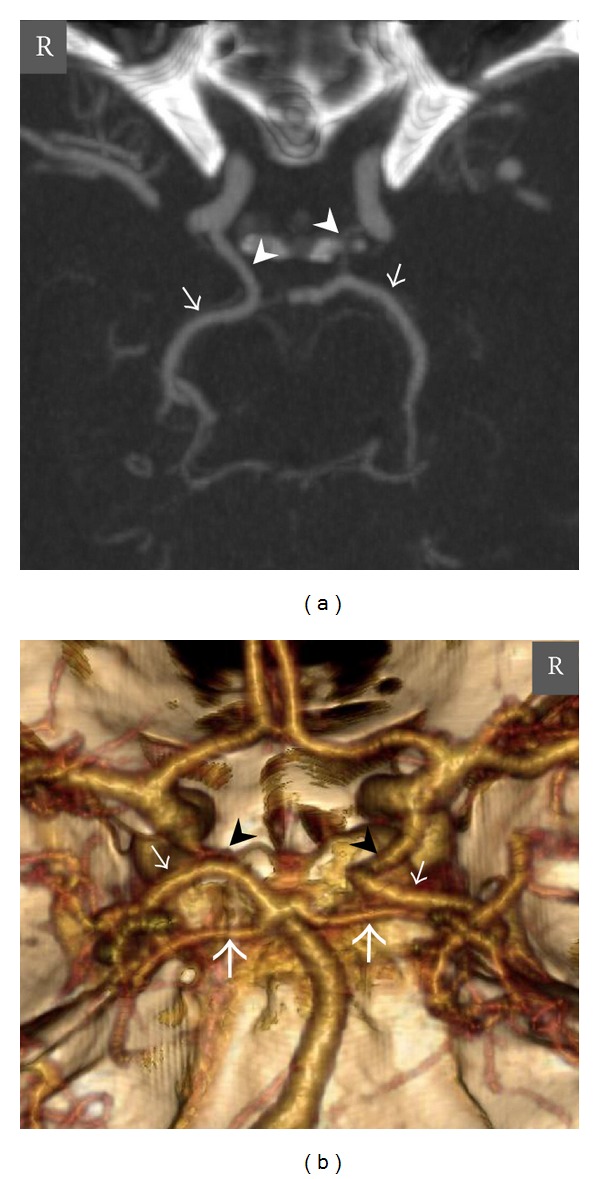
Axial MIP (a) and posteroanterior view VR (b) CT angiography images show a right PCA with fetal origin in a 42-year-old man. The P1 segment of the right PCA is agenetic, and there is a well-developed right PCoA that supplies the P2 and distal segments of the right PCA. The P1 segment of the left PCA is patent, and there is a thin calibrated left PCoA. *Thick short arrows *= right and left SCAs, *thin short arrows *= right and left PCAs, *arrowheads* = right and left PCoAs, and *R* = right.
